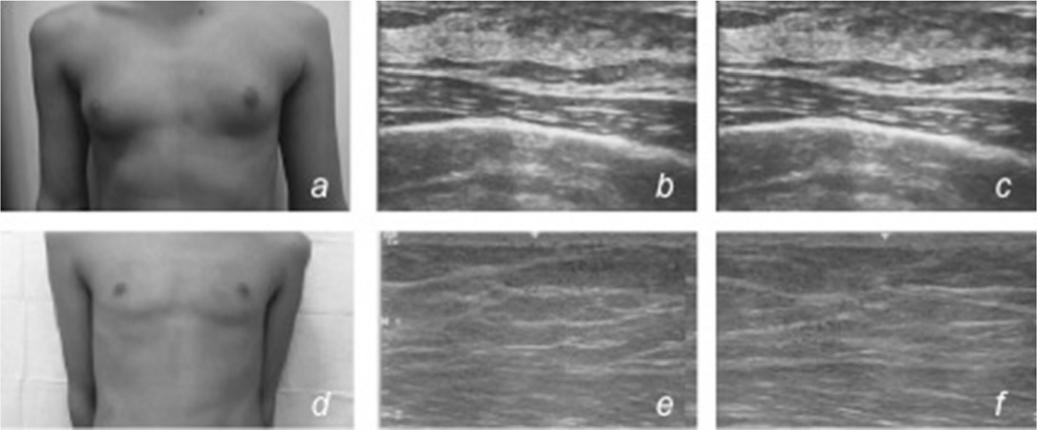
Patient 1. Clinical preoperative breast view (a) and breast ultrasound (b,c) scan showing bilateral gynecomastia. No solid nodular or cystic formations are present on either side; clinical postoperative breast view (d) and breast ultrasound (e,f) scan 1 year after surgery showing a total absence of glandular parenchyma, with muscle planes well represented.
